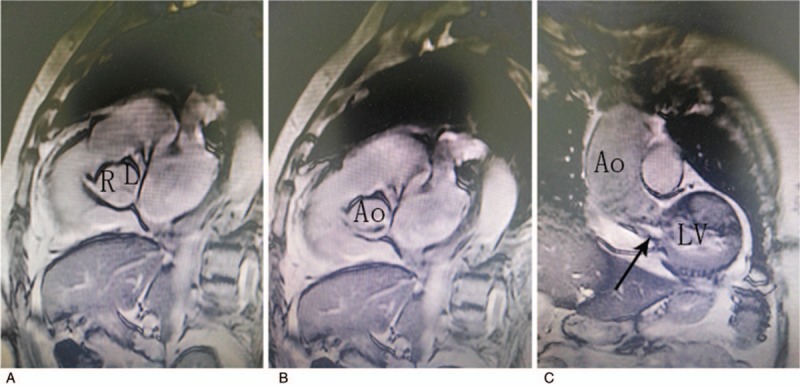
Magnetic resonance images. (A) Three leaflets were well closed when the aortic valve was closed. (B) The aortic valve was found with restricted opening, and the blood flow only pushed through the aortic noncoronary leaflet when the left ventricle was contracted. (C) The arrow shows that the white region was the high-velocity blood flow region when the left ventricle contracted, and only one leaflet (noncoronary leaflet) was pushed open.
